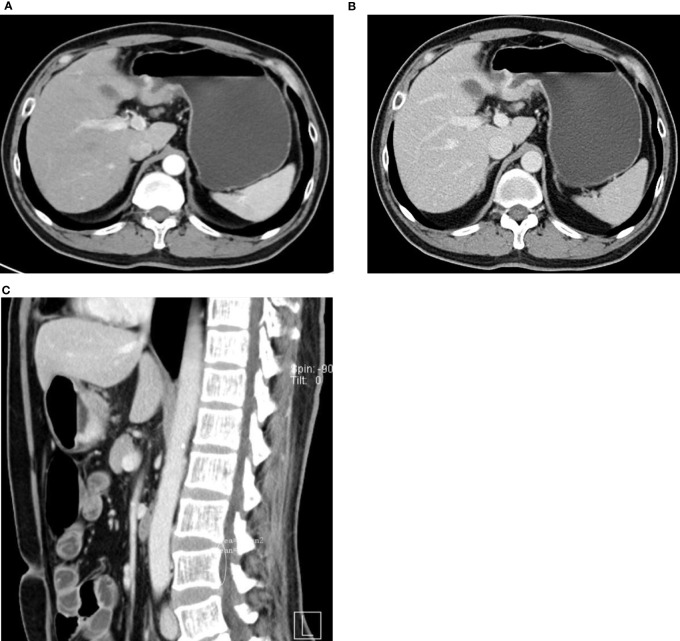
47-year-old man with HAS. (A–C) Thickened wall at the gastric antrum. Nodular/irregular protrusion with an obscure surrounding fat layer. Evident continuous enhancement of lesion on enhancement CT.
